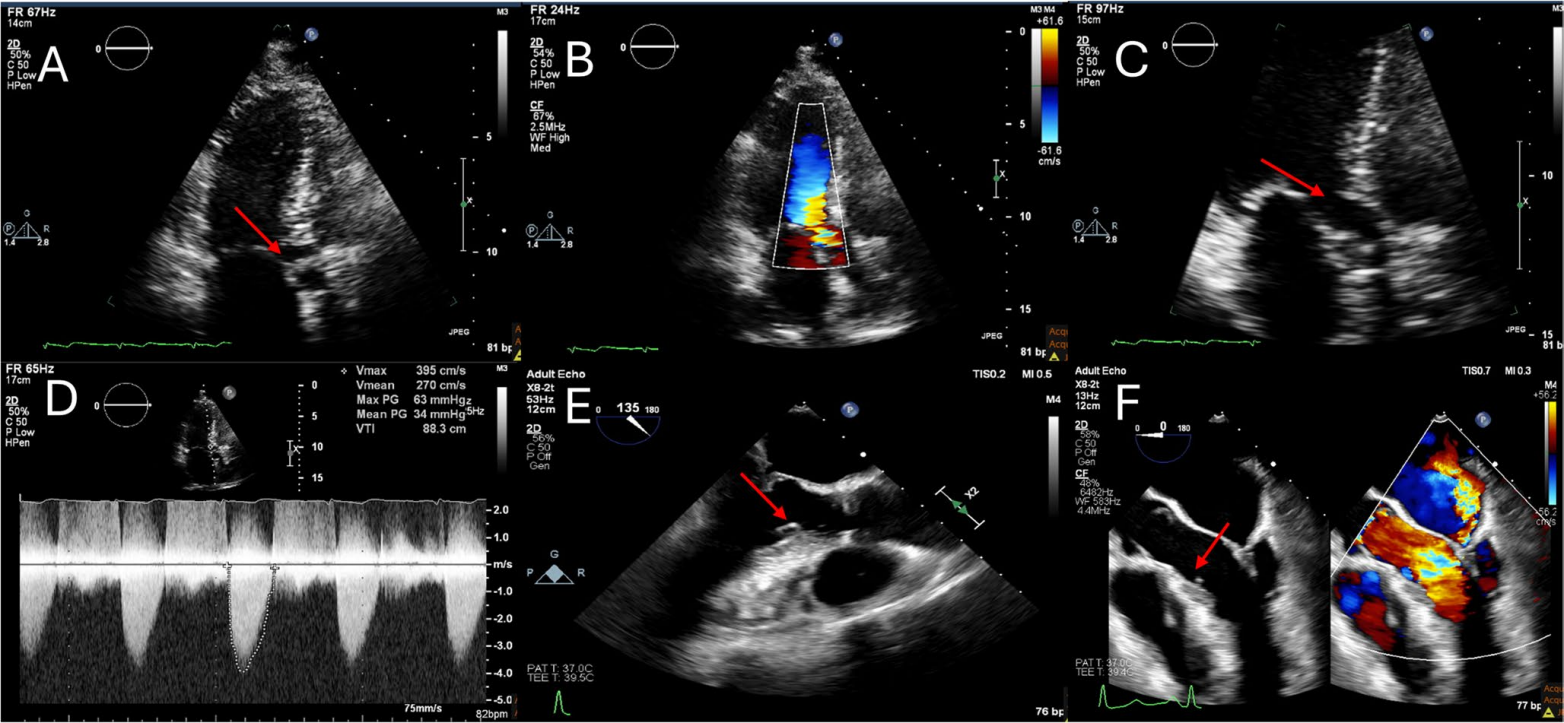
(A) Subaortic membrane (arrow) visualized on 2D echocardiography. (B) Flow acceleration at the level of the membrane (C) Subaortic membrane (arrow) visualized on 2D echocardiography with continuous wave Doppler showing a fixed obstructive profile with peak velocity of 4.0 cm/s (D) mean gradient of 34mmHg (E) Transesophageal echocardiography showing a subaortic membrane (arrow) on 2D (F) Another patient transesophageal echocardiography showing subaortic membrane (arrow) with flow acceleration by color Doppler at the level of the membrane along with significant mitral regurgitation
